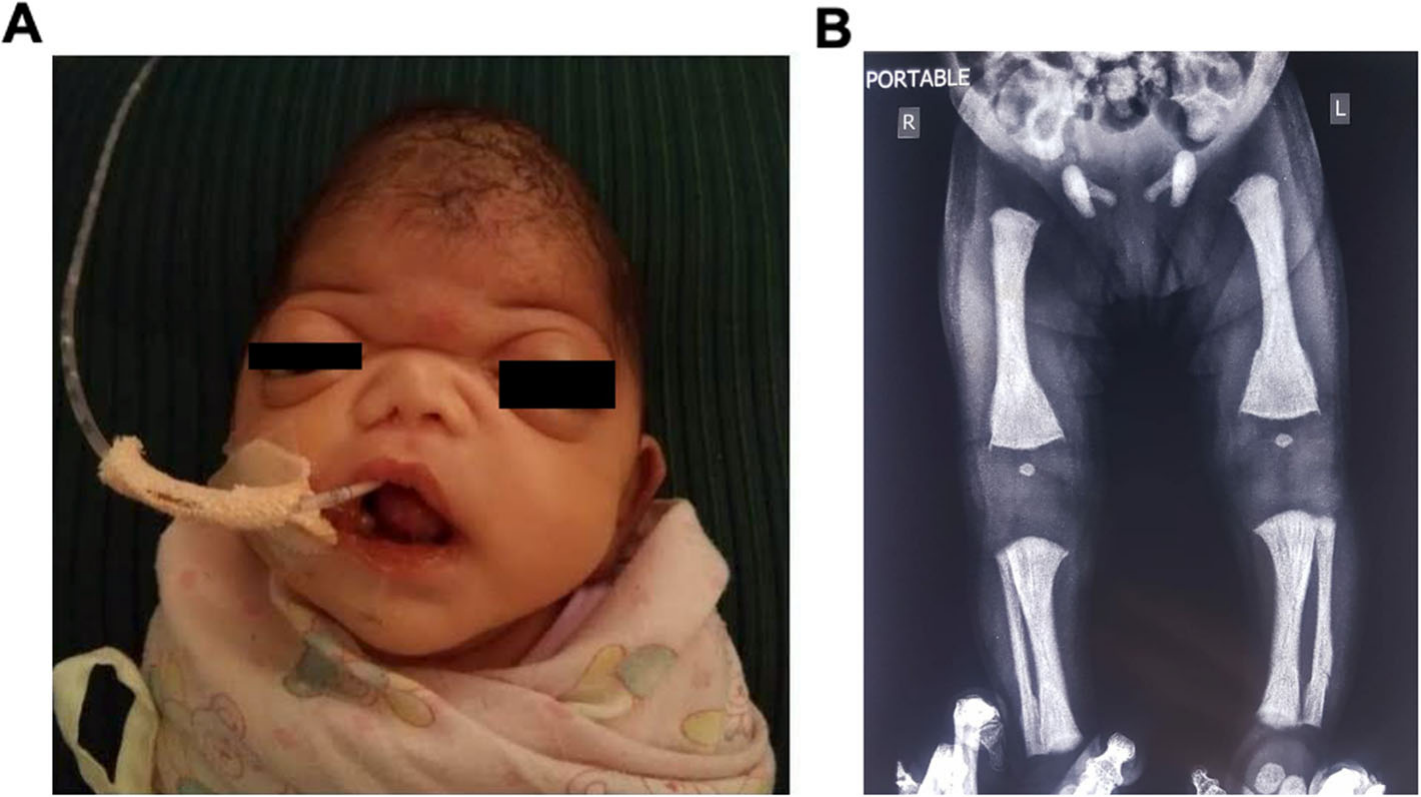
Clinical and radiographic profile of the child. a Facial photograph depicting gross dysmorphism. b Frontal view of the pelvic radiograph depicting generalized osteosclerosis, poor corticomedullary differentiation, microfractures in left femur and left fibula
